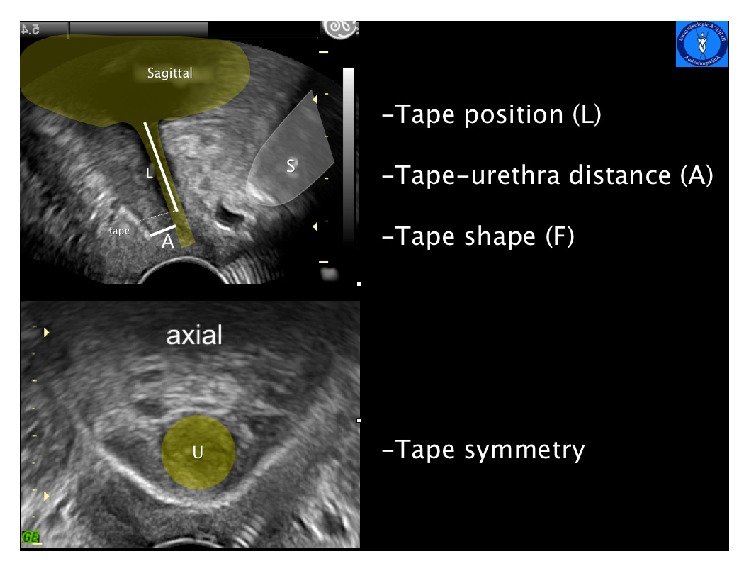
Pelvic floor-ultrasound images in a sagittal plane (above) and axial plane (below). S: symphysis pubis, U: urethra, L: tape position in relation to the urethra length, and A: shortest distance of the tape from the LSM complex of the urethra.
